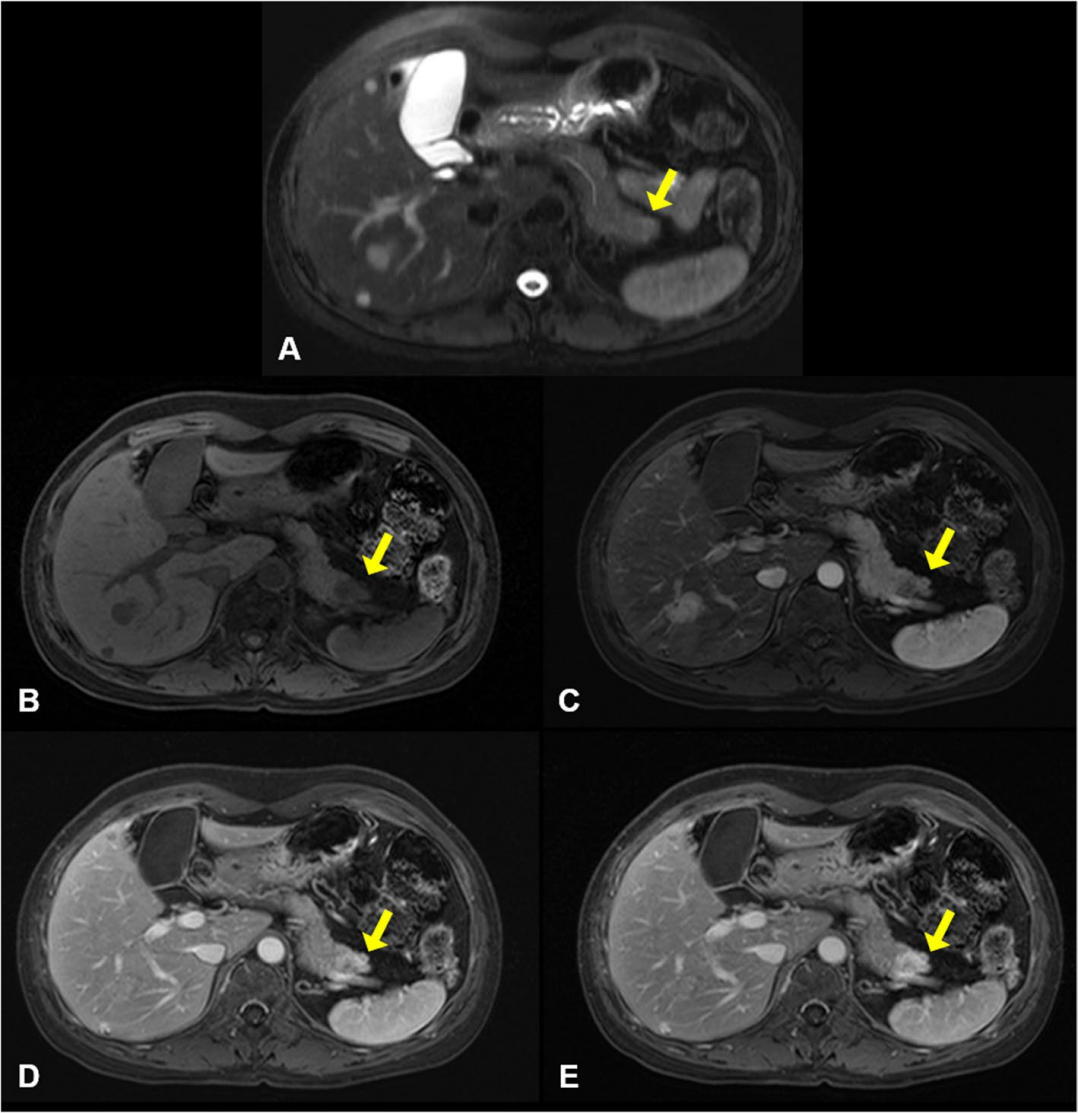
Abdominal magnetic resonance imaging of pancreatic mass. A In the pancreatic tail, a subtle high-signal intensity lesion was identified on T2‑weighted images. Dynamic gadoxetic acid-enhanced magnetic resonance images of the pancreatic mass showed a low signal intensity mass on pre-contrast images (B) and low contrast enhancement on the arterial phase images (C), followed by delayed contrast enhancement on portal phase (D) and delayed phage (E) images. The yellow arrow indicates the mass
